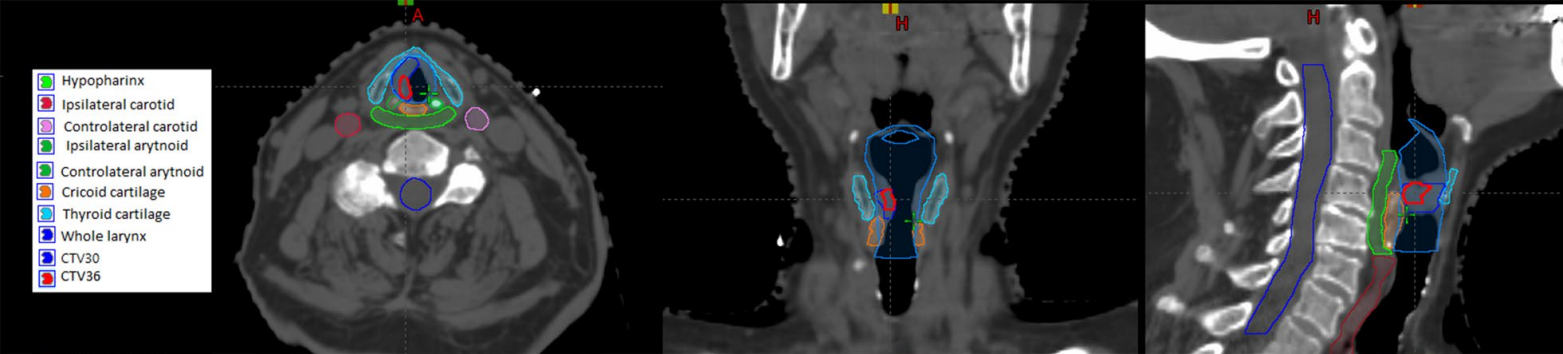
Target and organs at risk as delineated on CT images
